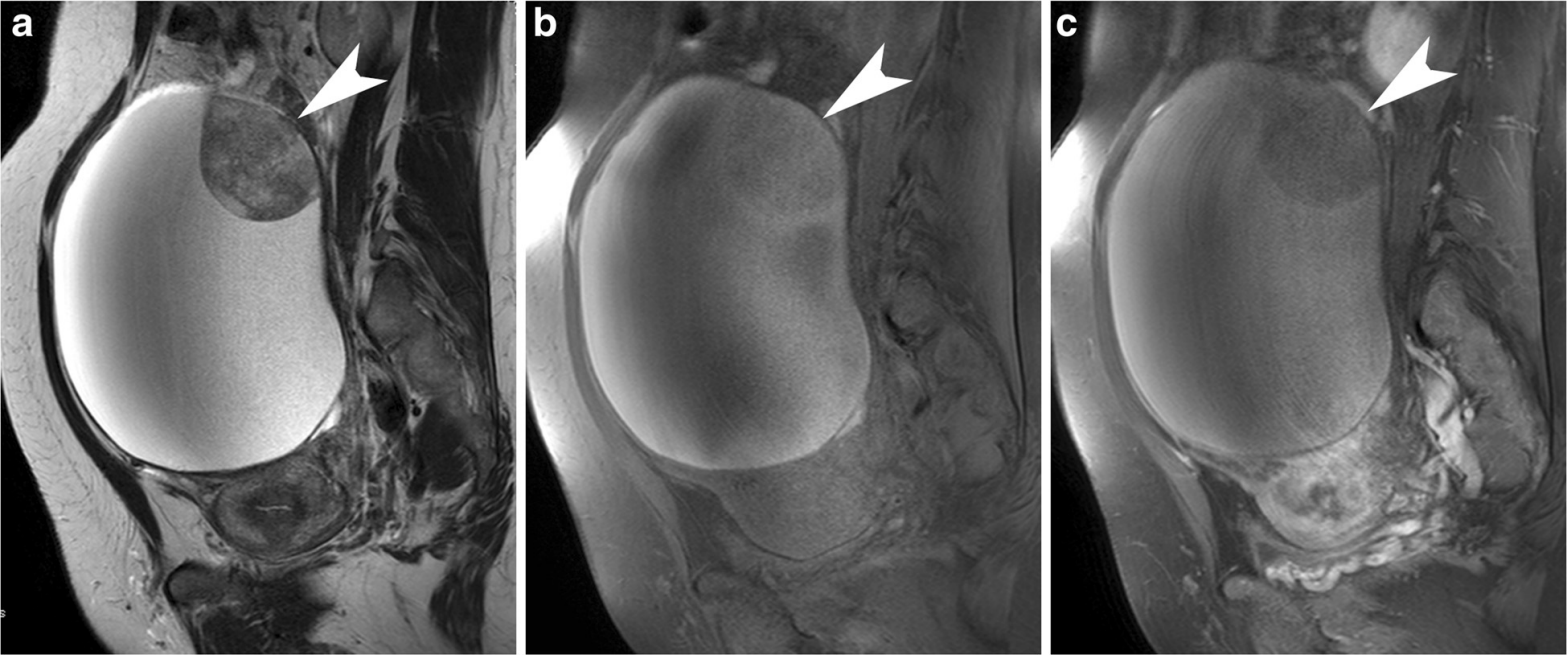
A case with clear cell carcinoma. A solitary large unilocular cystic mass with a mural nodule is demonstrated on the uterus on sagittal T2-weighted image (a). The lack of adipose tissue between the mass and the uterus suggest adhesion, which evoke co-existing endometriosis. In addition, the content of the cyst shows high signal intensity on sagittal fat-saturated T1-weighted image is another evidence of hemorrhagic material included within an endometriotic cyst (b). After administration of contrast material, the mural nodule is weakly enhanced (arrow heads, b: sagittal T1-weighted image, c: contrast-enhanced and fat-saturated T1-weighted image)
